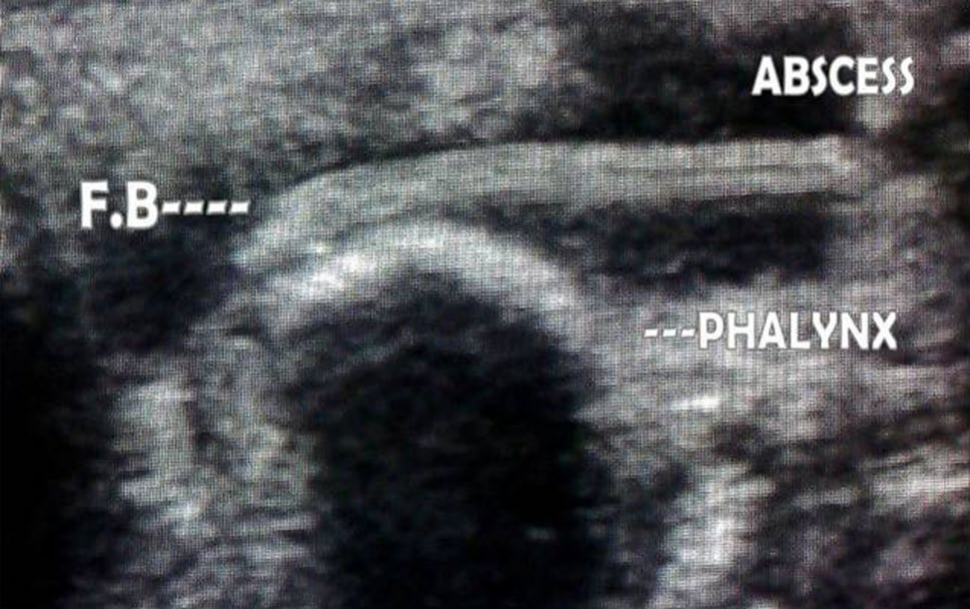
Ultrasound of hand showing foreign body with abscess formation on dorsum of hand
